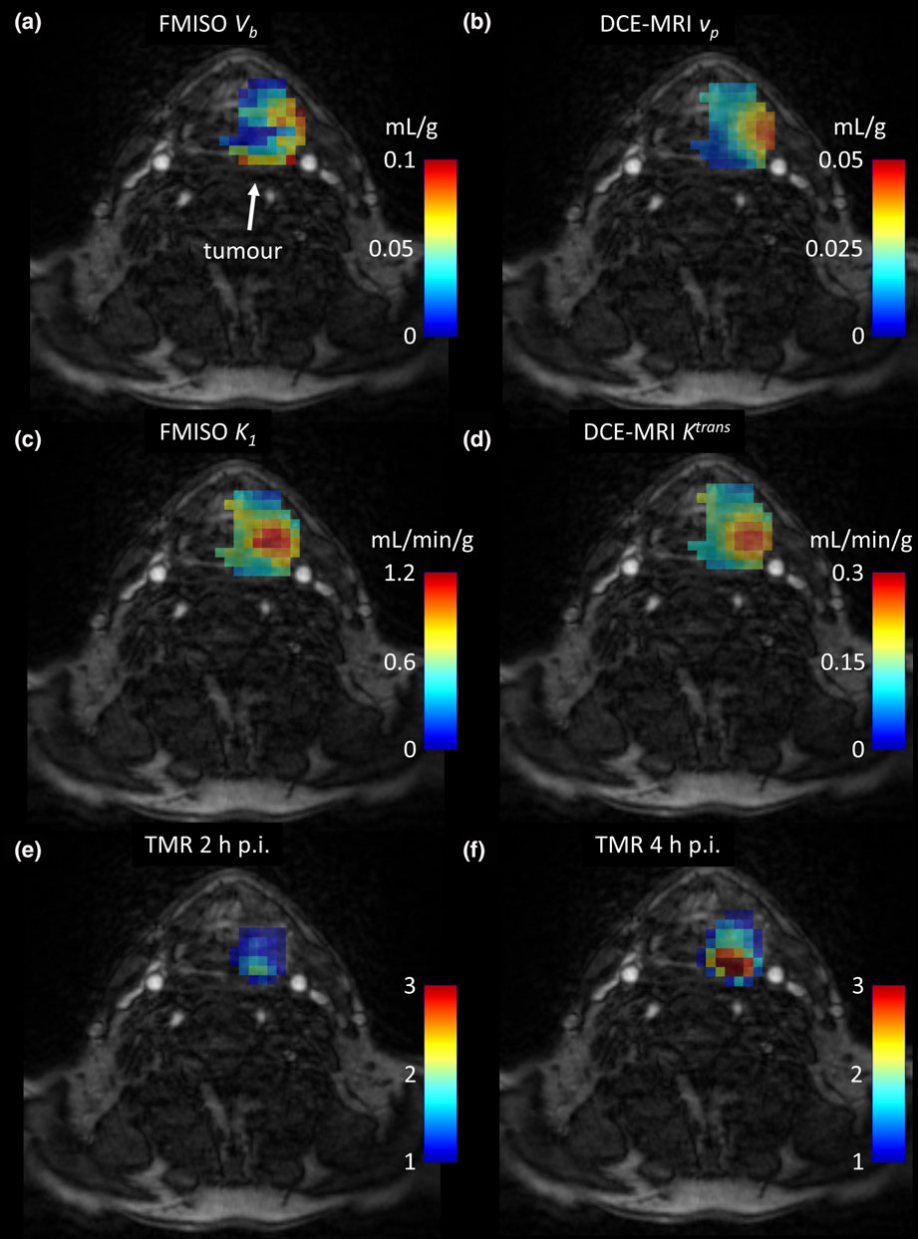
Contrast‐enhanced MRI images of the patient P4, overlaid with the FMISO
